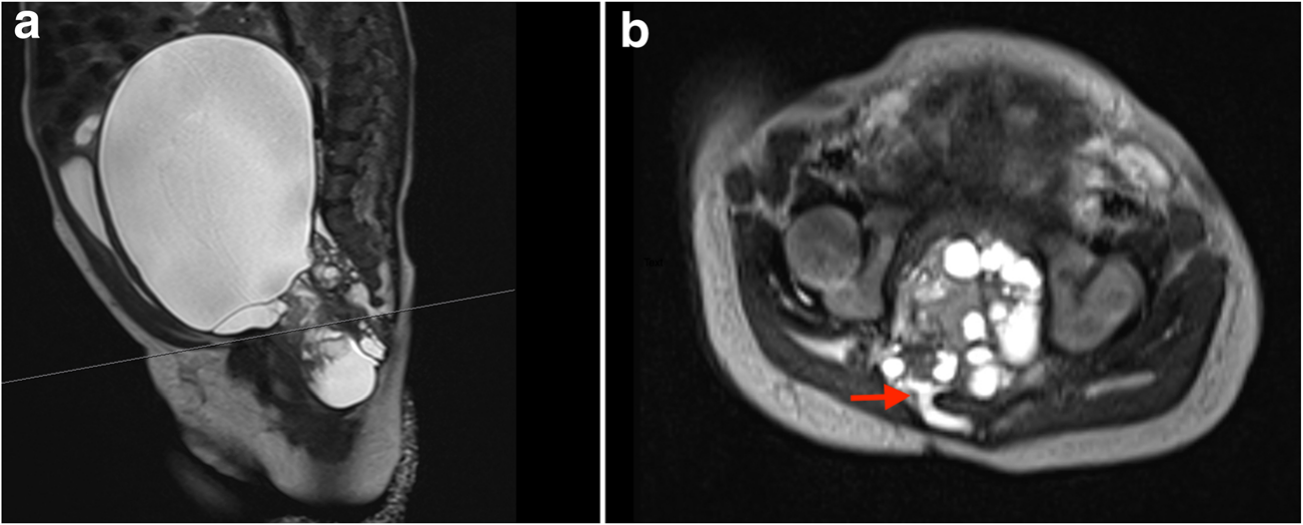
Preoperative, T2-weighted sagittal (left), and axial (right) MR images of the abdomen and pelvis, demonstrating a large cystic mass within the pelvis abutting the sacrum and coccyx. A small posterior extension of the pelvic mass, extending through an enlarged sacral foramen, is observed posterior to the sacral elements (arrow)
